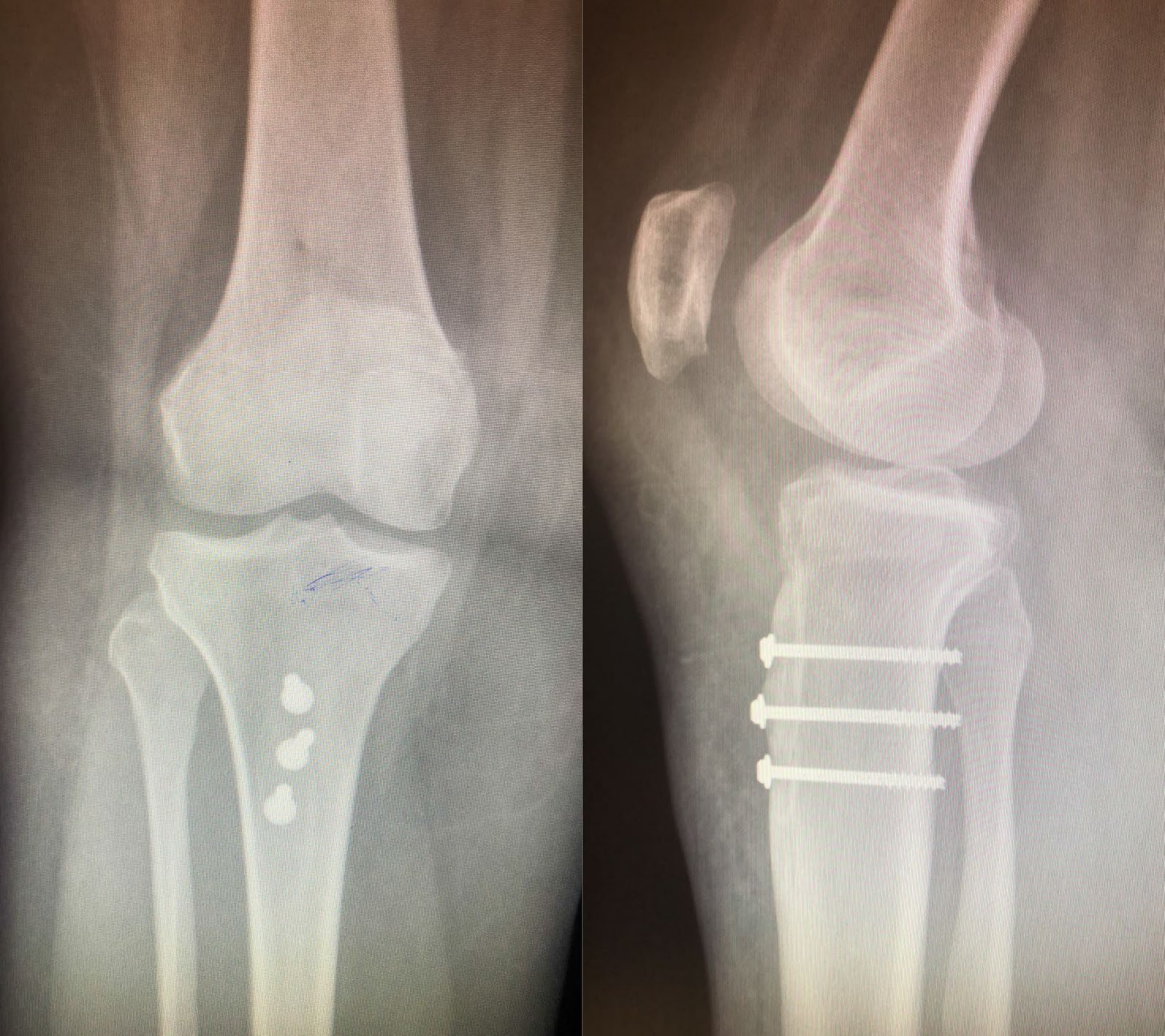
Antero-posterior and lateral post-operative plain radiographs demonstrating fixation of tibial tuberosity transfer
